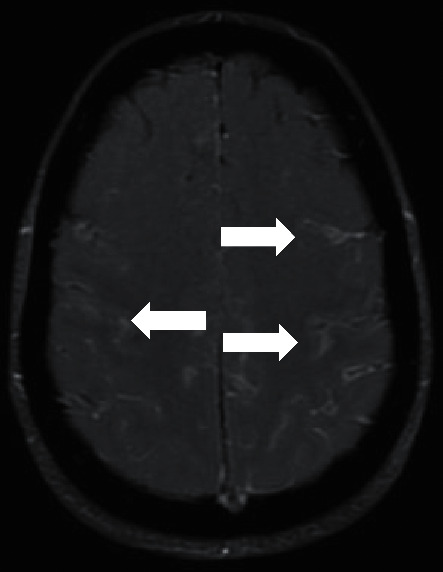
Brain MRI: T1, after gadolinium administration; arrows: leptomeningeal enhancement.
